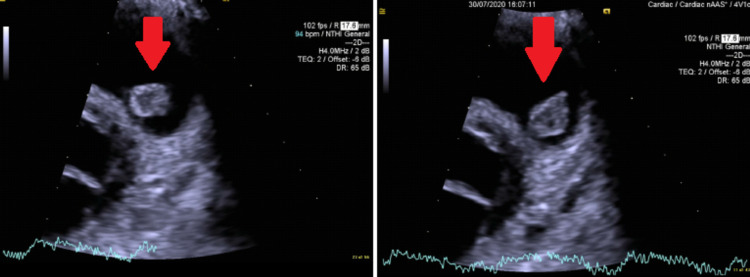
Echocardiogram showing a 1.8 cm vegetation attached to the pulmonary valve
